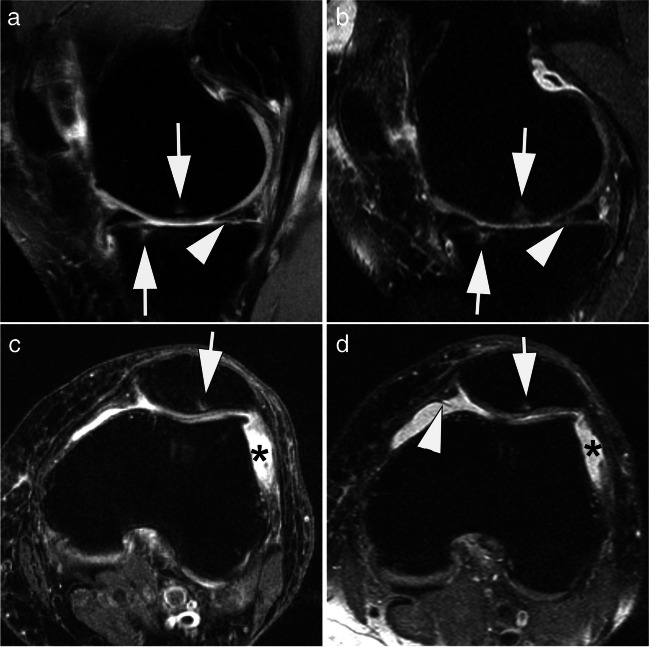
Comparison of a 1.0-T small-bore extremity system with a standard 1.5 T large-bore MRI for assessment of knee osteoarthritis. A Standard 1.5-T proton density-weighted fat-suppressed image shows small subchondral bone marrow lesions (BMLs) at the central medial femur and the anterior medial tibia (arrows). In addition, there is a horizontal oblique degenerative tear of the posterior horn of the medial meniscus (arrowhead). B At 1.0-T extremity MRI, BMLs (arrows) and tear (arrowhead) are visualized with similar image quality. C Axial 1.5-T proton density-weighted fat-suppressed image shows a small BML at the lateral patella (arrow) and joint effusion (asterisk). D Axial 1.0-T image shows BML (arrow) and effusion (asterisk) in similar fashion. In addition, there is a small medial patellar plica (arrowhead). TA: 3:53 min (A), 4:44 min (B), 2:45 min (C), 2:59 min (D)
